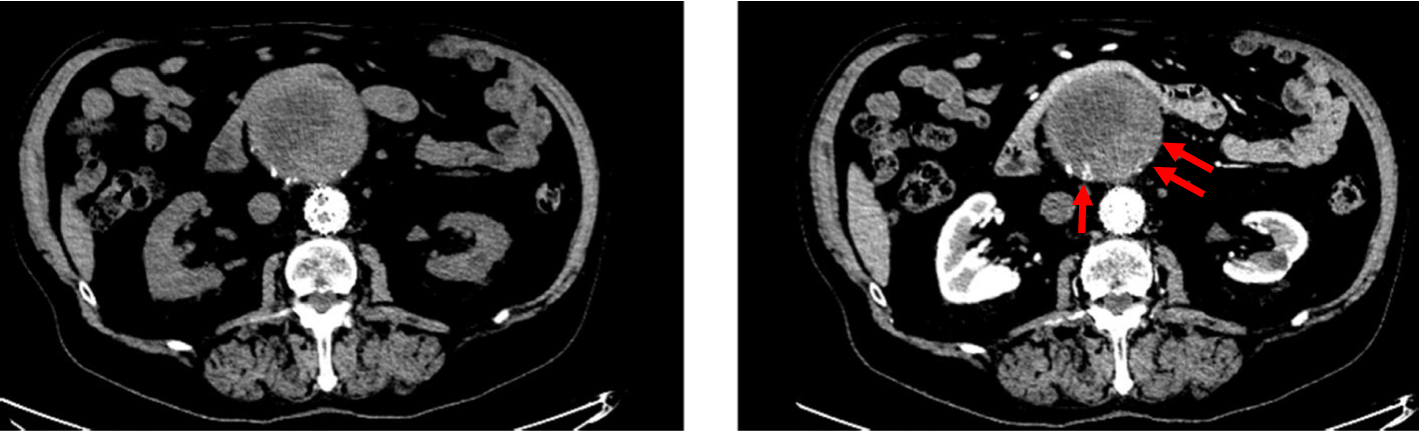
Pre and post contrast CT images of Patient 1 following embolisation of small T2EL which demonstrates enhancement of peripheral sac thrombus. Tiny foci of contrast opacification along the aortic wall (arrows) may represent vasa vasora
